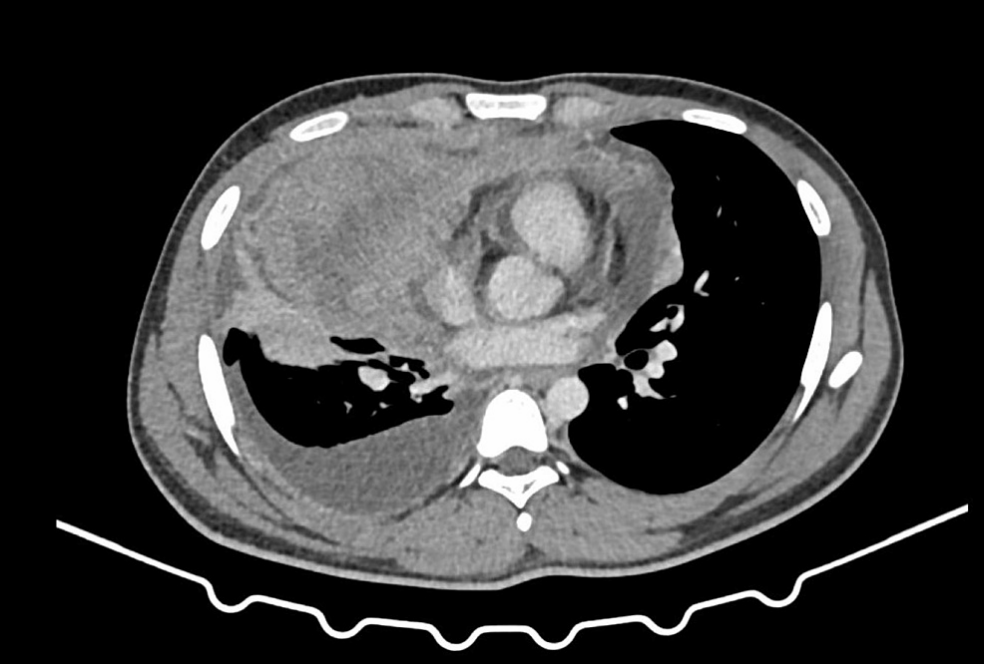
Contrast-enhanced computed tomography scan of the thorax: axial cut, mediastinal window showing a well-defined, heterogeneously enhancing soft tissue density lesion measuring ~ 7.85 x 16.5 x 10.0 cm (AP x TR x CC) noted in the anterior mediastinum predominantly on the right side with extensions to the left. Few non-enhancing areas are noted within the likely necrosis, with no calcifications noted.
